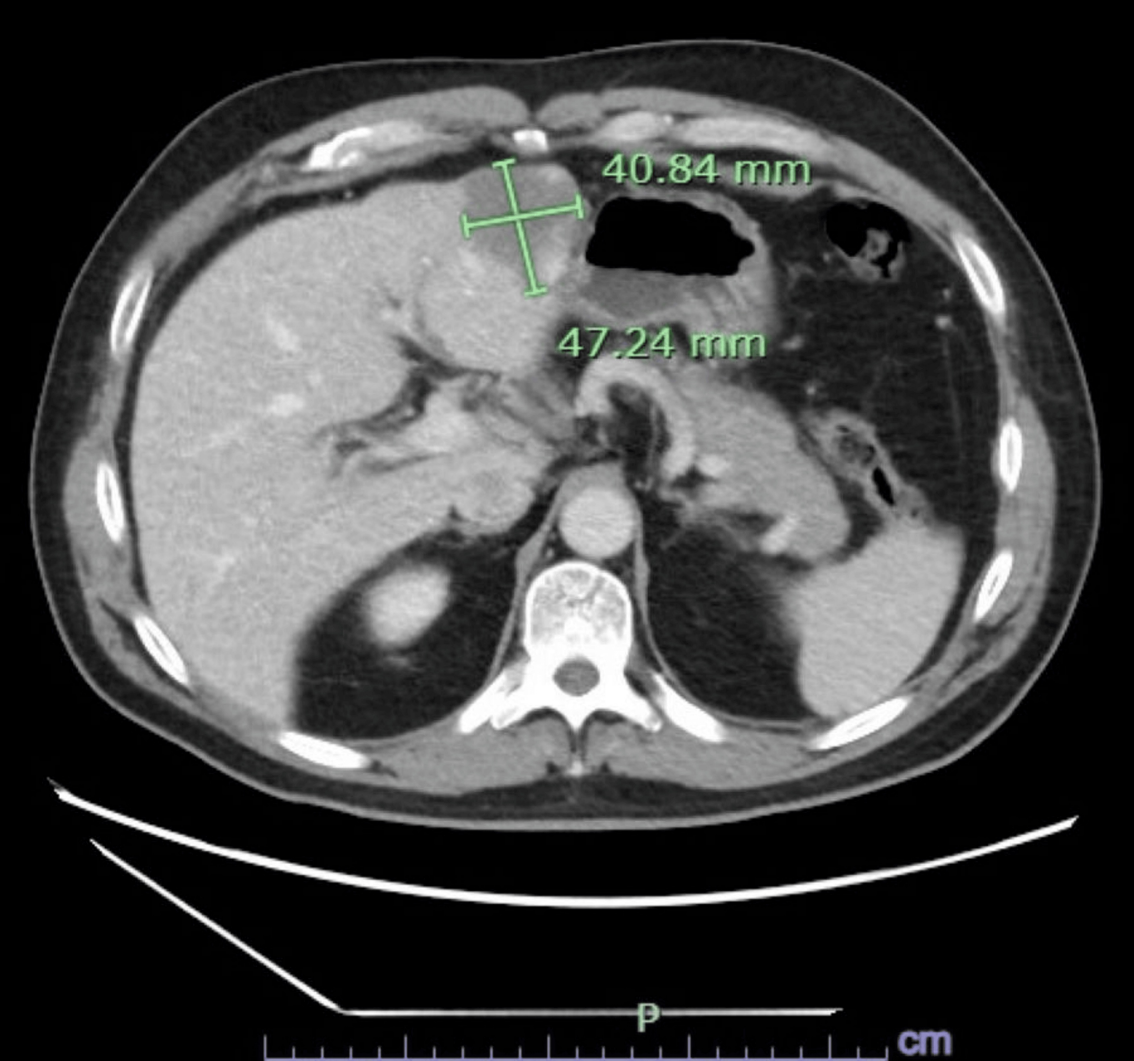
Contrast-enhanced CT of the abdomen demonstrating a hepatic lesion concerning for malignancy and a subtle nodular liver contour suggestive of early cirrhosis.
